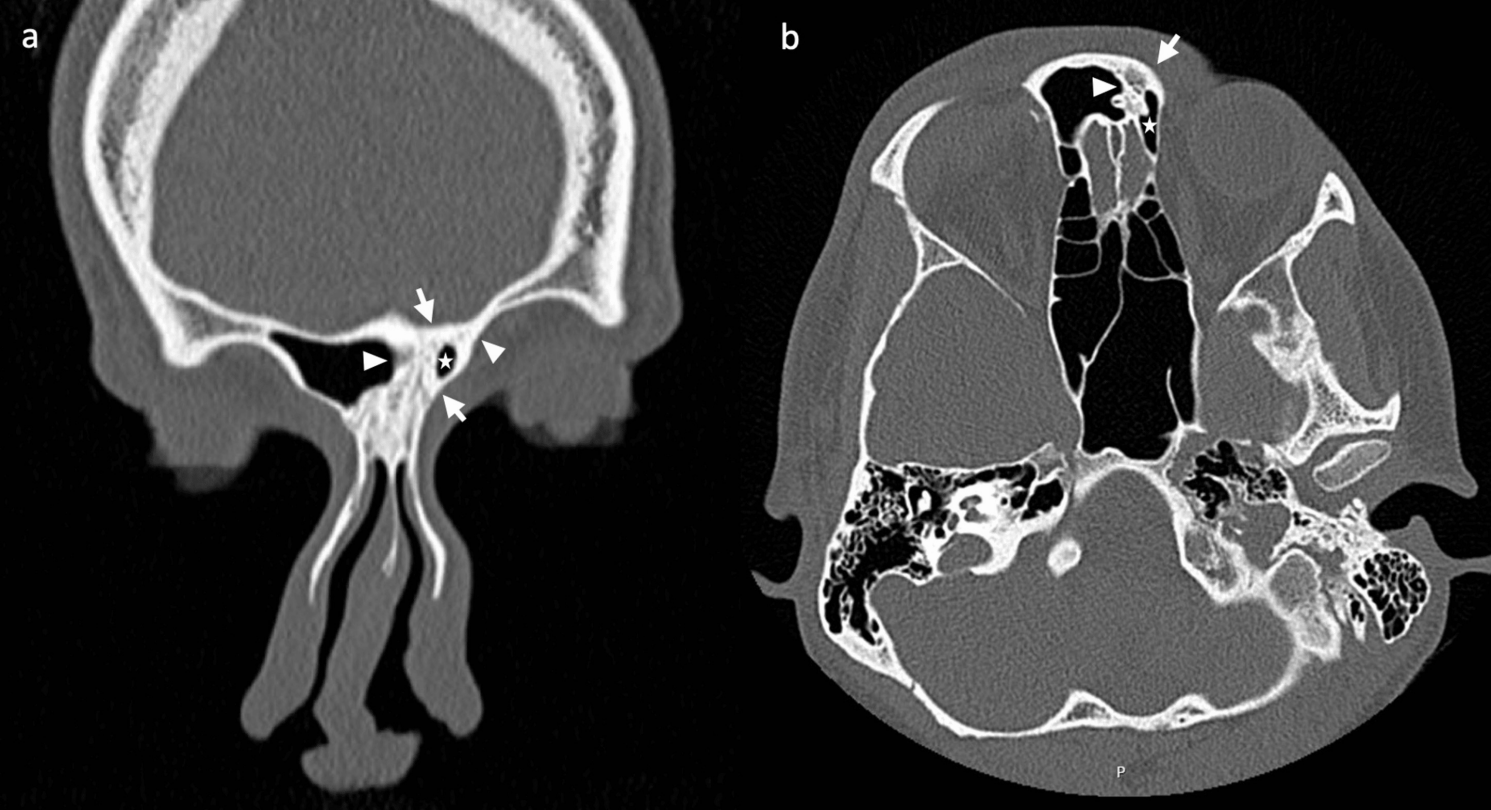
Coronal (a) and axial (b) slices of computed tomography scans demonstrating reduced left frontal sinus volume (star) secondary to retraction of the inter-frontal sinus septum to the left (arrowhead) and upwards displacement of floor of the left frontal sinus (arrowhead) with evidence of hyperostosis of the frontal bone (arrows).
